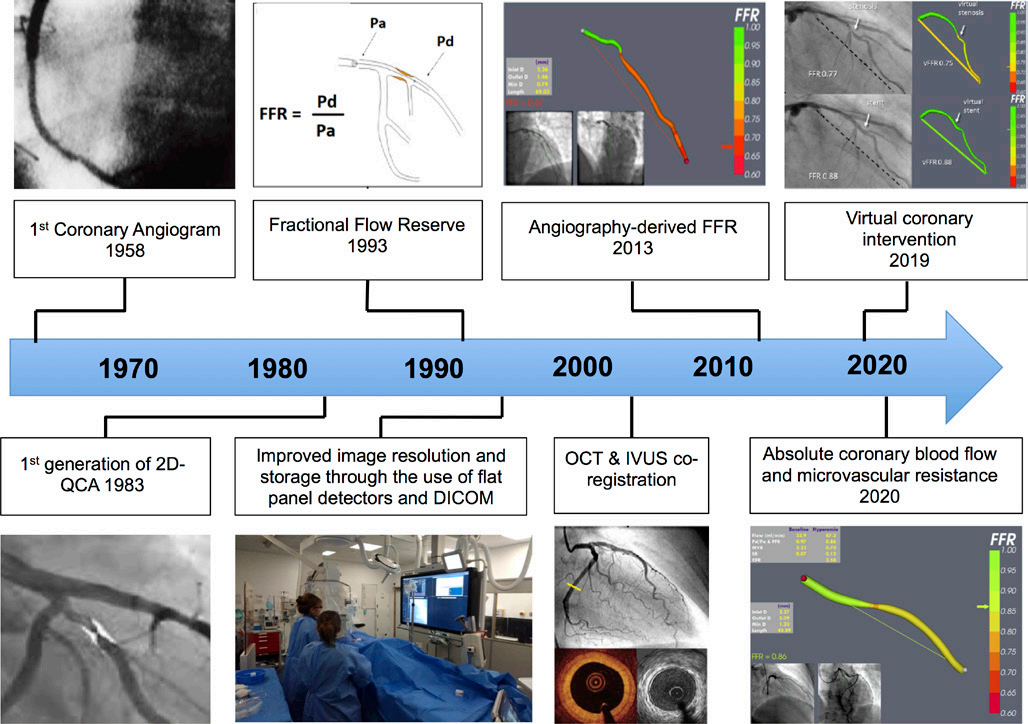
Milestones in the history of diagnostic coronary angiography. 2D, two-dimensional; FFR, fractional flow reserve; IVUS, intravascular ultrasound; OCT, optical coherence tomography; Pa, aortic pressure; Pd, pressure distal to stenosis; QCA, quantitative coronary angiography. DICOM, Digital Imaging and Communications in Medicine
